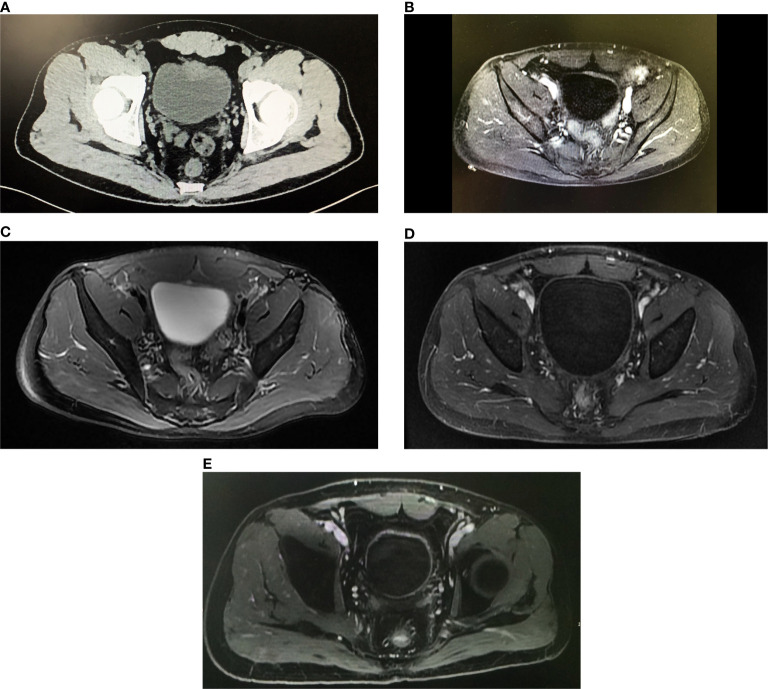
Imaging of the patient over the course of therapy. (A) The axial pelvic CT image before treatment demonstrated a mass was about 3.0 cm arising from the anterior wall of the bladder and the perivesical fat was invaded. (B) After two cycles of neoadjuvant therapy, the axial image from dynamic contrast-enhanced pelvic MRI indicated the thickness of enhanced anterior wall of the bladder decreased. (C) After four cycles of neoadjuvant therapy, the axial T2W image from pelvic MRI showed the light thickening of the anterior wall was persisting, but no node or tumor was found. (D) After concurrent chemoradiotherapy plus pembrolizumab, the axial contrast-enhanced MRI on arterial phase showed the bladder was normal with no sign of tumor recurrence. (E) The axial contrast-enhanced MRI on arterial phase still showed no tumor in the bladder at the last follow-up on February 20, 2021.
